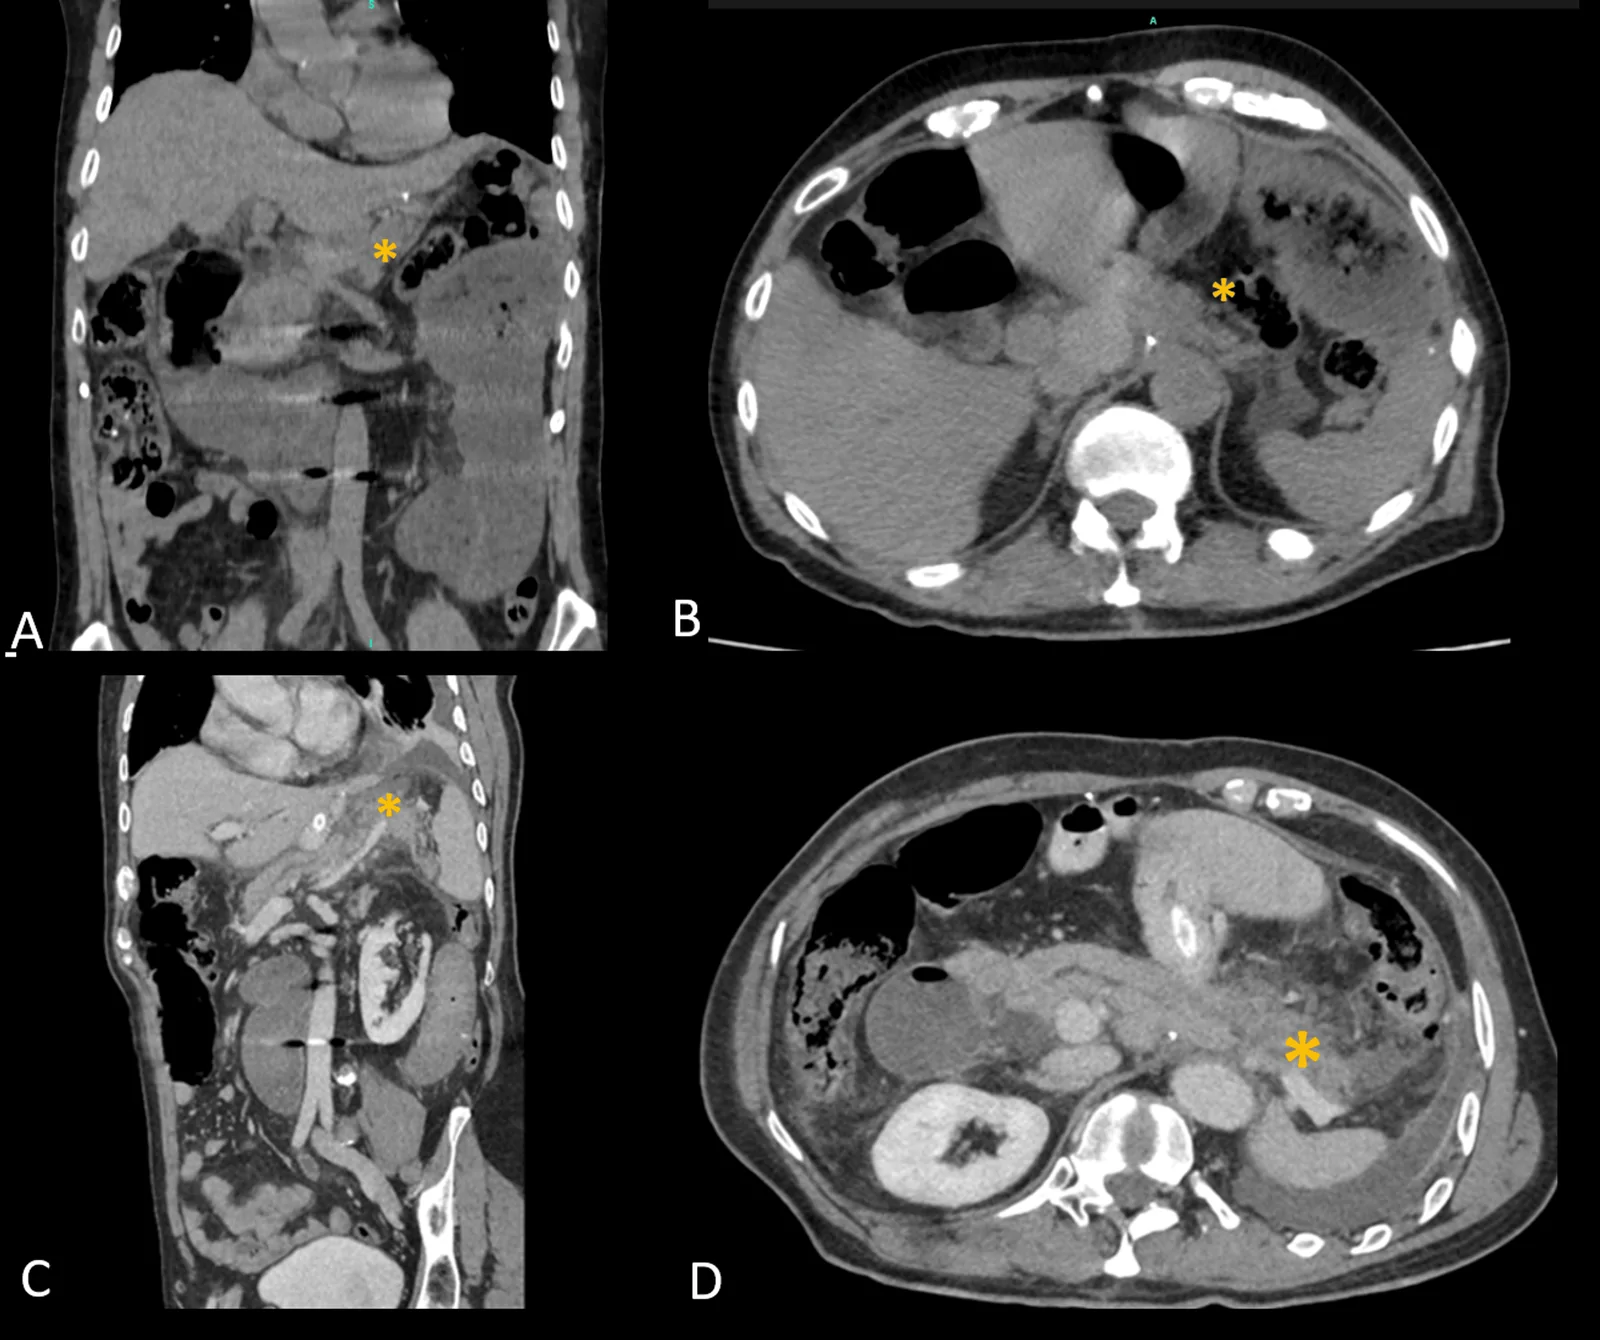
Contrast-enhanced abdominal CT demonstrating the evolution of pancreatic findings. A and B: Abdominal CT obtained approximately 24 hours after symptom onset showing focal pancreatic hypoenhancement (yellow asterisk). C and D: Repeat CTs were obtained approximately 72 hours after symptom onset, showing progression to extensive non-enhancing pancreatic parenchyma (yellow asterisk), consistent with necrotizing pancreatitis. Coronal (A), coronal oblique (C), and axial (B and D) images are shown.
